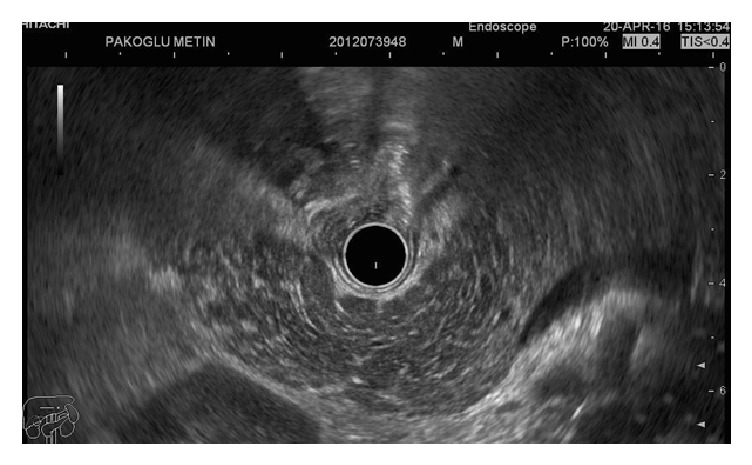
A radial endosonographic image for indeterminate for chronic pancreatitis.
